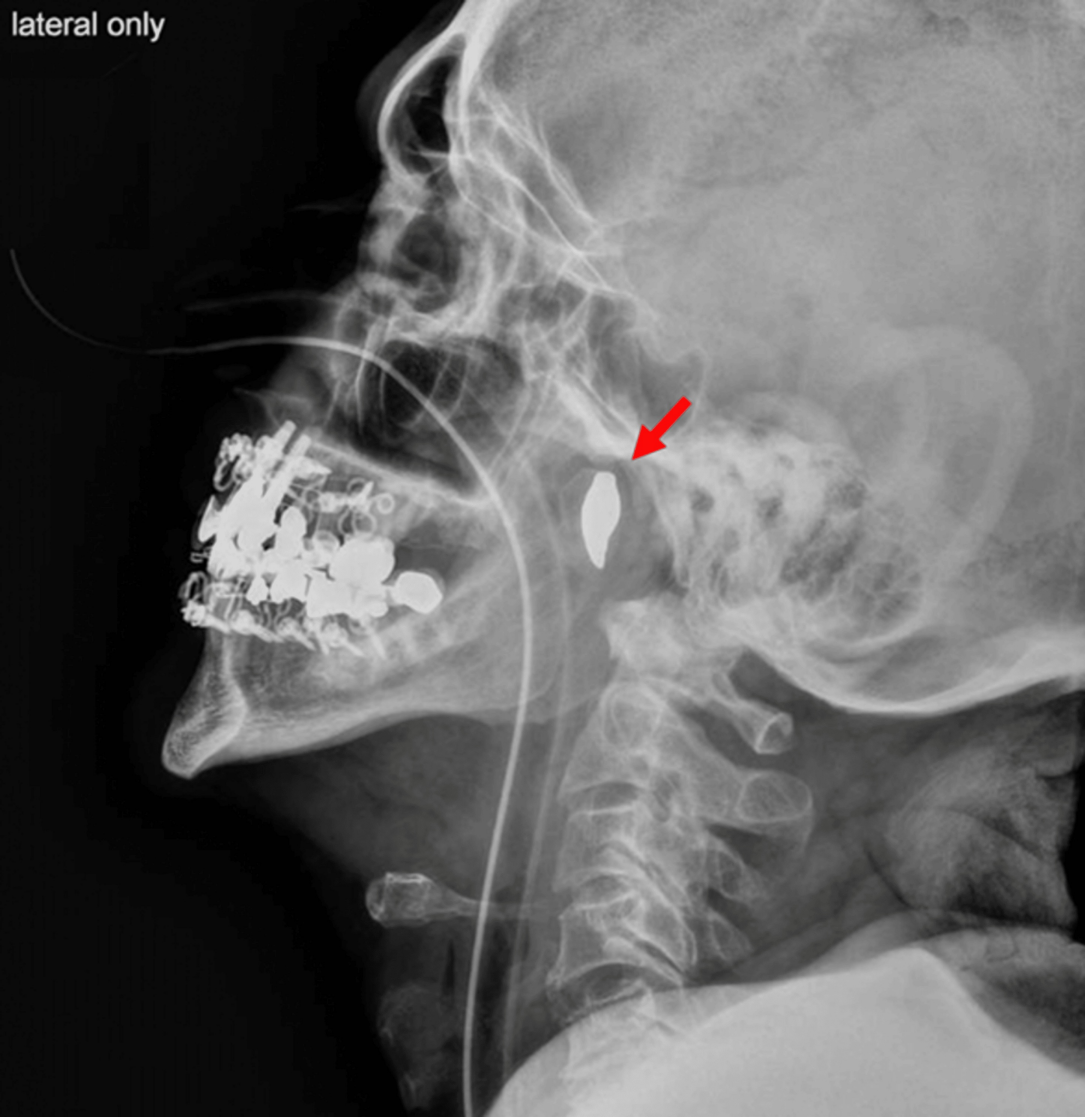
Lateral radiograph of the neck demonstrating a solitary radiopaque tooth in the nasopharynx
